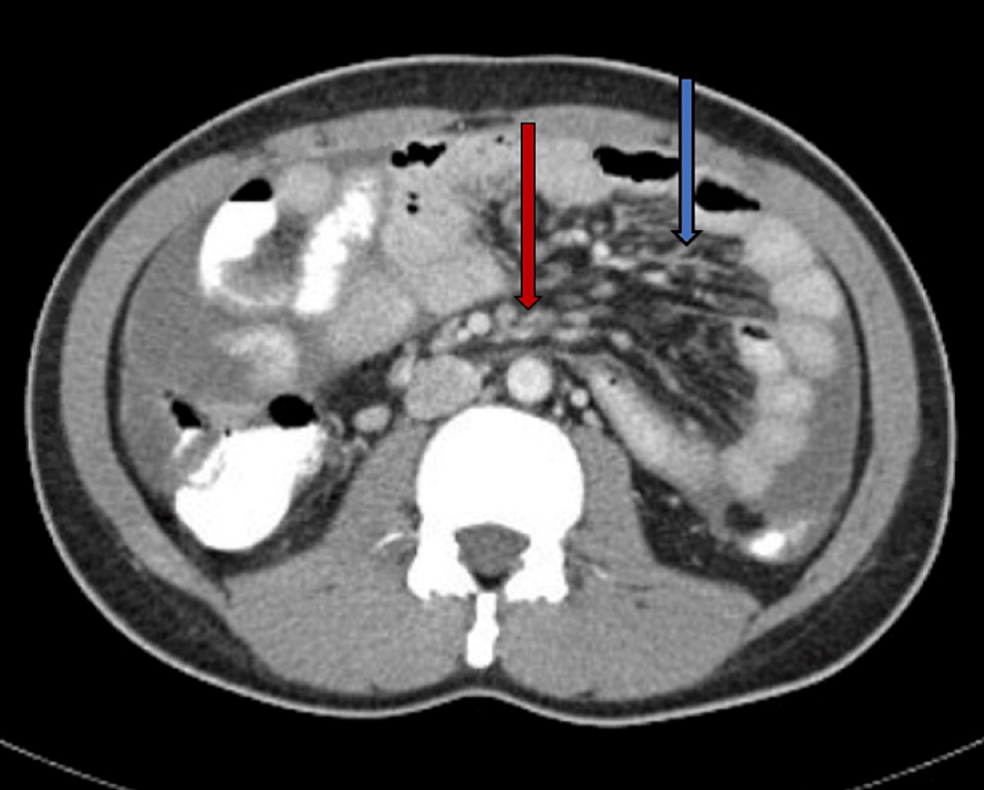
CT scan of the abdomen showing mesenteric thickening (blue arrow) and mesenteric nodularity (red arrow).
